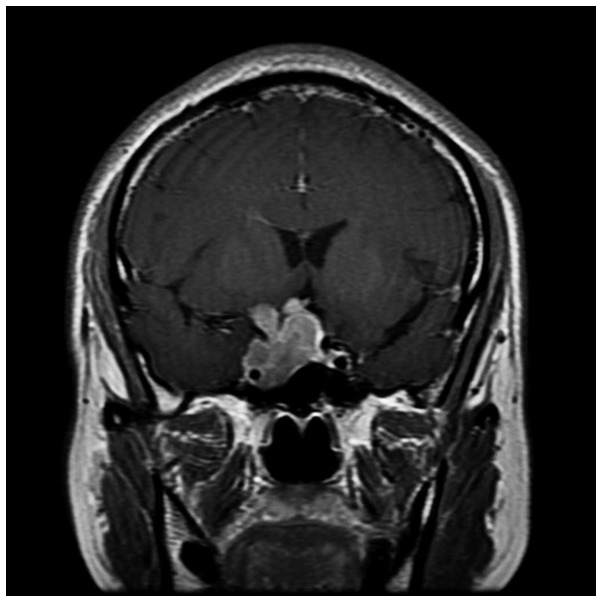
MRI showing an irregular abnormal signal of ~3.7×2.1 cm in size, with marked heterogeneous enhancement on the contrast MRI, which affected the right cavernous sinus and surrounded the right internal carotid artery. The pituitary stalk was compressed to the left, which caused obliterations of suprasellar cistern. MRI, magnetic resonance imaging.
